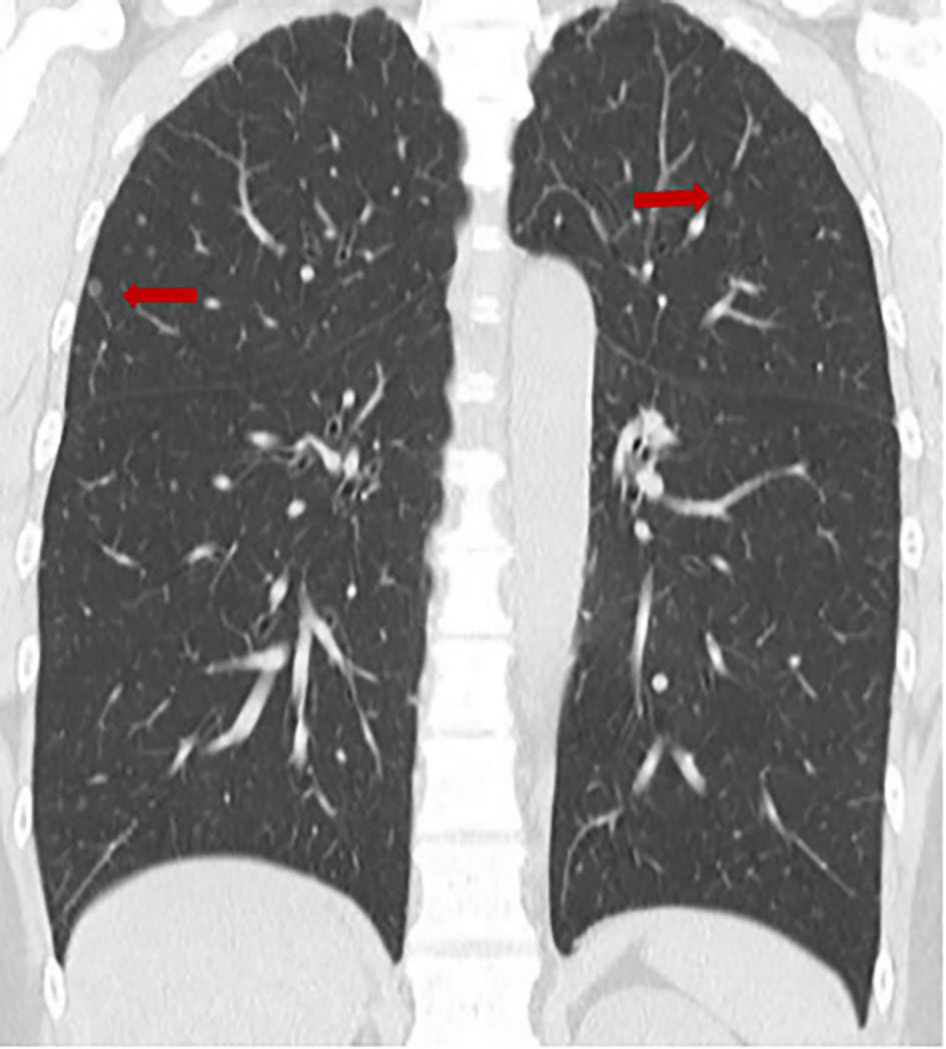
Chest CT demonstrating innumerable 1–2‐mm micronodules diffusely distributed in both lungs, predominantly peripheral/subpleural (arrows).
